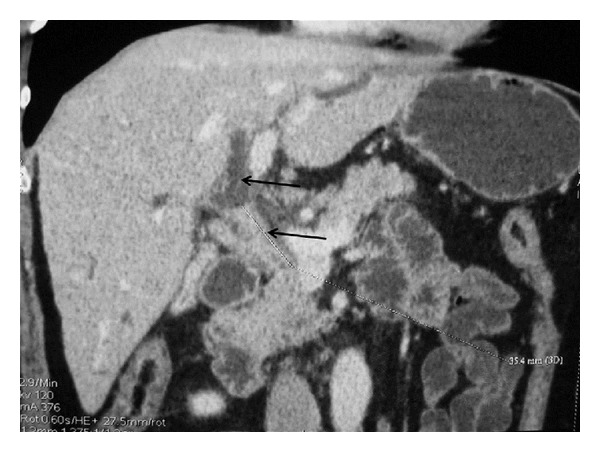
Abdominal CT scan: circumferential thickening of the bile duct with dilatation of the biliary tree upstream.
